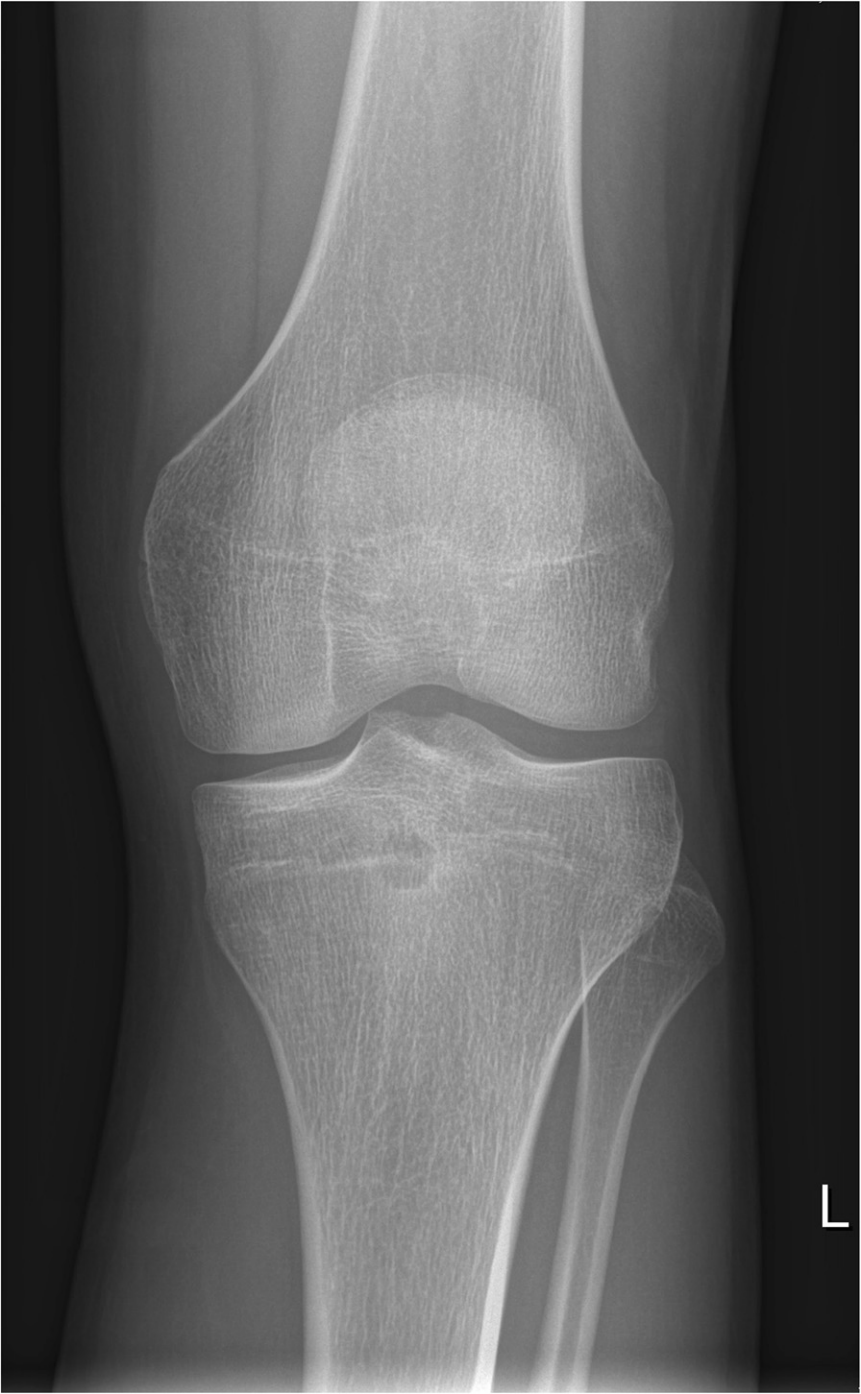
X-ray of the left knee (anteroposterior view), taken at the first out-patient presentation
